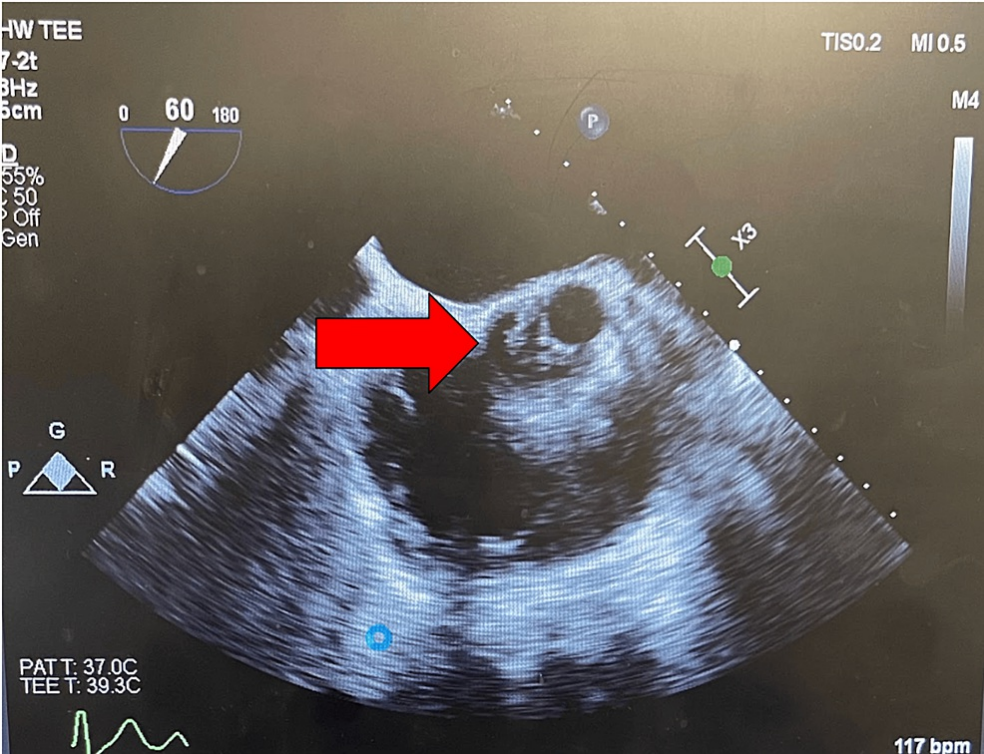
Extensive vegetation and aortic regurgitation shown on TEE TEE: transesophageal echocardiogram
